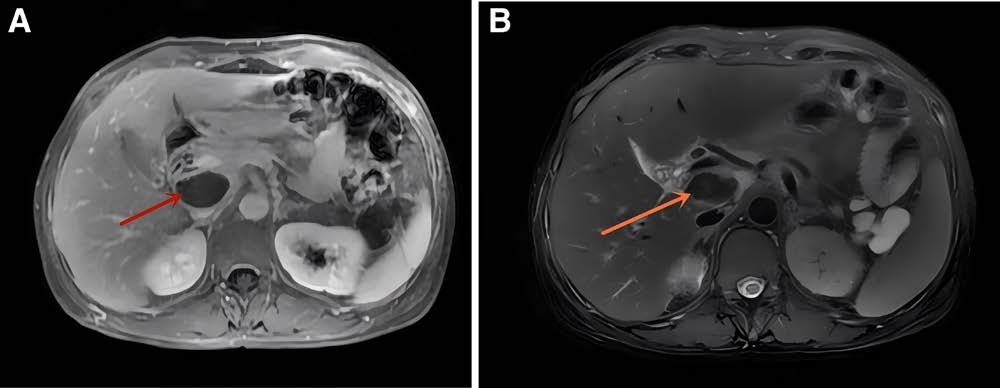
(A and B) MRI plain scan and enhancement were performed in the hepatic and biliary area showed abnormal signals in the portal vein and inferior vena cava space were considered as hematoma. MRI = magnetic resonance imaging.
